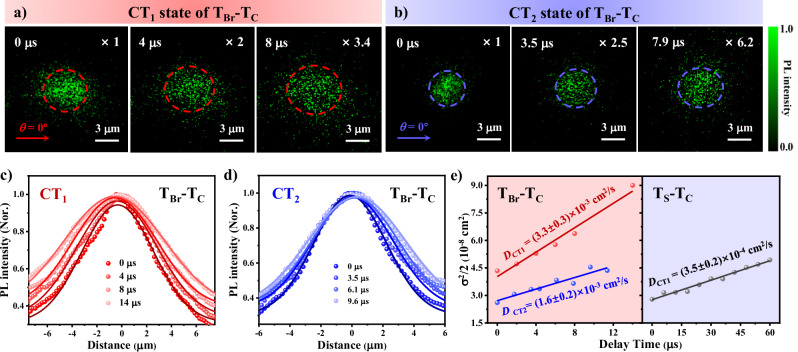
Imaging and modeling of CT exciton transport. Time-dependent TADF intensity images of a CT_1_ and b CT_2_ excitons in T_Br_-T_C_ collected at 670-750 nm and 490-550 nm, respectively. Scale bars are 3 μm. The dash lines represent the exciton distribution obtained from the Gaussian fittings. Normalized 1D TADF intensity profiles of c CT_1_ and d CT_2_ excitons in T_Br_-T_C_ extracted from the *θ*= 0° direction in panels a and b, respectively, at the indicated delay times. The solid lines are their Gaussian fittings. e The determination of TADF-related exciton diffusion coefficient by the linear fitting of 1D time-dependent Gaussian variances ($${\sigma }^{2}\left(t\right)$$) along the *θ* = 0° direction.
